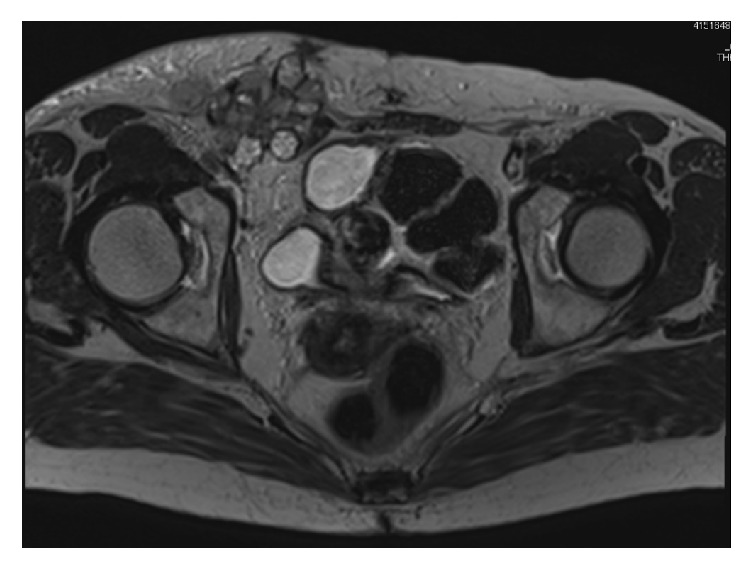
T2-weighted axial MR image, showing a multilobular mass consisting of cystic and solid components at the right inguinal site.
